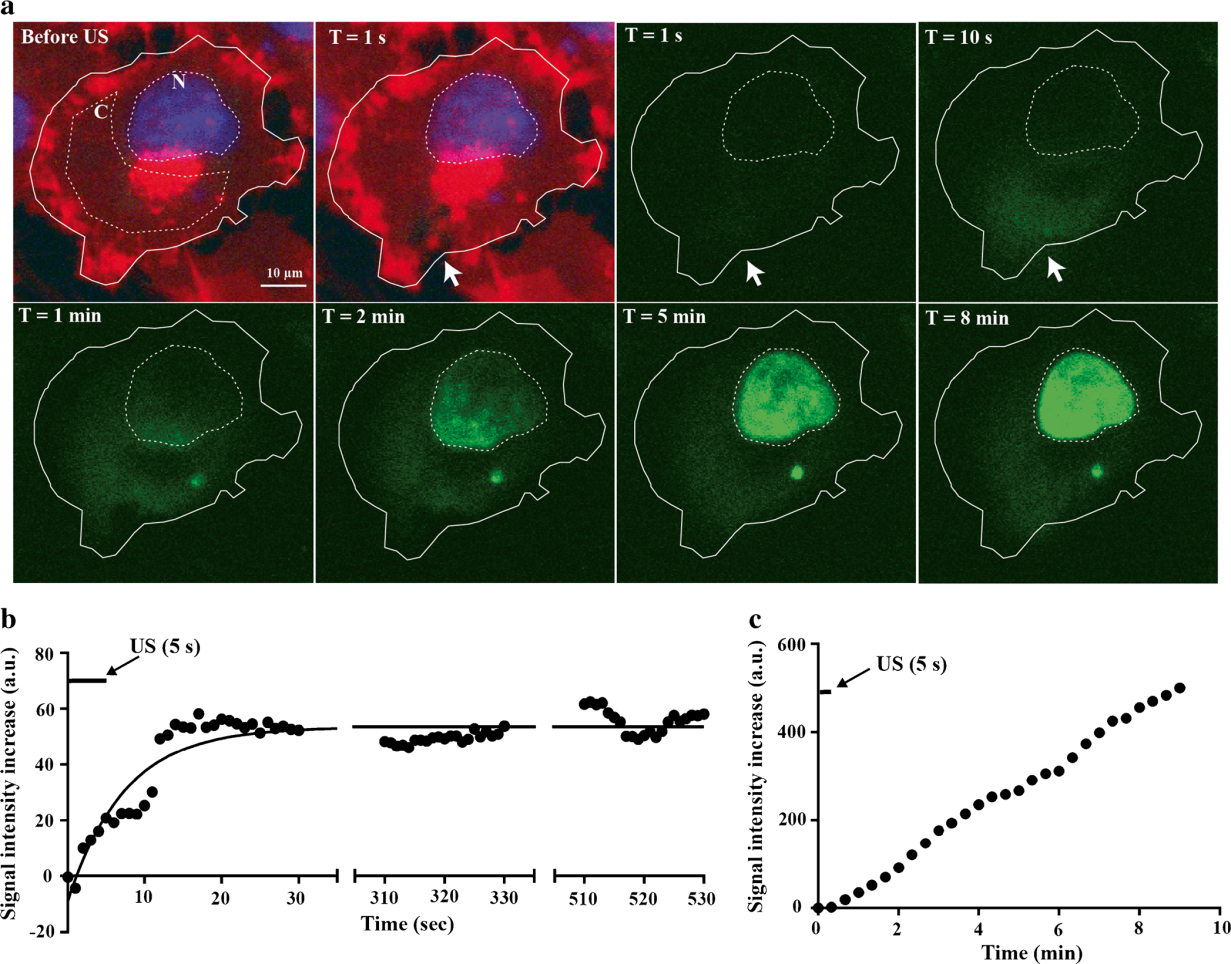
USMB-induced SYTOX Green uptake. a Time series of confocal images. Cell membrane and nucleus were labeled in *red* and *blue* to show the cell morphology before ultrasound was turned on and the pore created by ultrasound (*white arrow*). SYTOX Green uptake is monitored over the next 8 min (shown in *green*). Two regions of interest were drawn in the cell to analyze the SYTOX Green signal in these compartments: *C* marks the cytosolic region of interest, and *N* marks the nucleus. Ultrasound was turned on for 5 s at *T* = 0. b Signal intensity time curve of SYTOX Green in the cytosolic region of interest of the BLM cell shown in (a). c Nuclear signal intensity time curve of SYTOX Green in the same cell. For the colored version of the figures, the reader is referred to the online manuscript.
